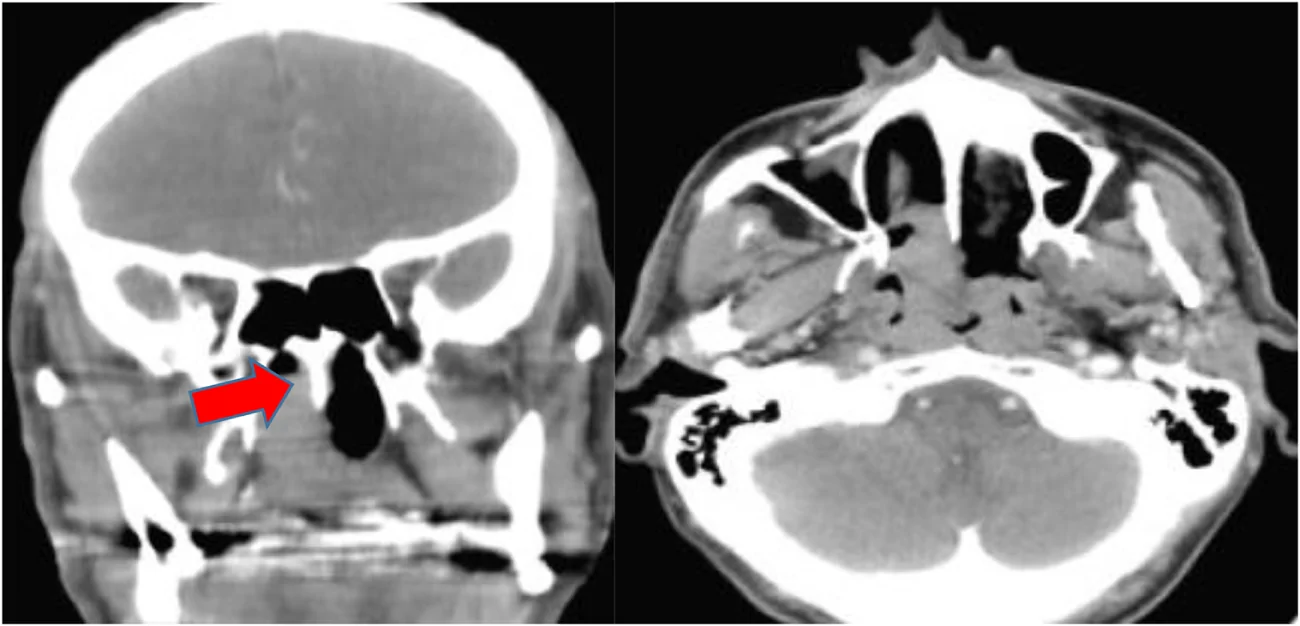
Contrast-enhanced coronal and axial CT images showing a homogeneous, intermediate-density lesion extending into the nasal floor and reaching the nasopharynx.
